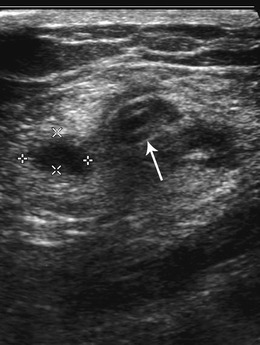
Tunnel infection seen in a patient with abdominal wall erythema and pain on ultrasound with a small hypoechoic collection adjacent to the catheter which is seen as parallel hyperechoic lines (*arrow*) within the anterior abdominal wall
